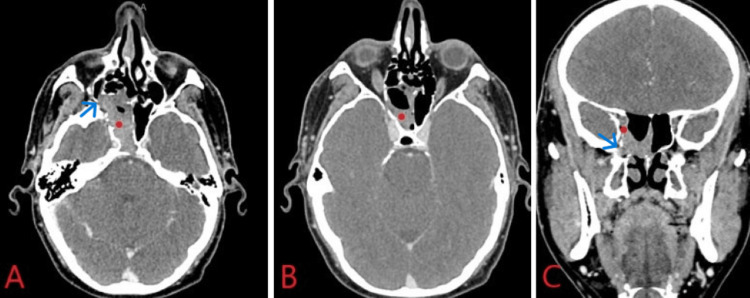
CT scan of sinuses in the axial (A, B) and coronal (C) planes after completion of induction chemotherapy. Noted extension of the mass to the PPF (blue arrow), with a lack of regression from the right maxillary and sphenoid sinuses (red dot).
